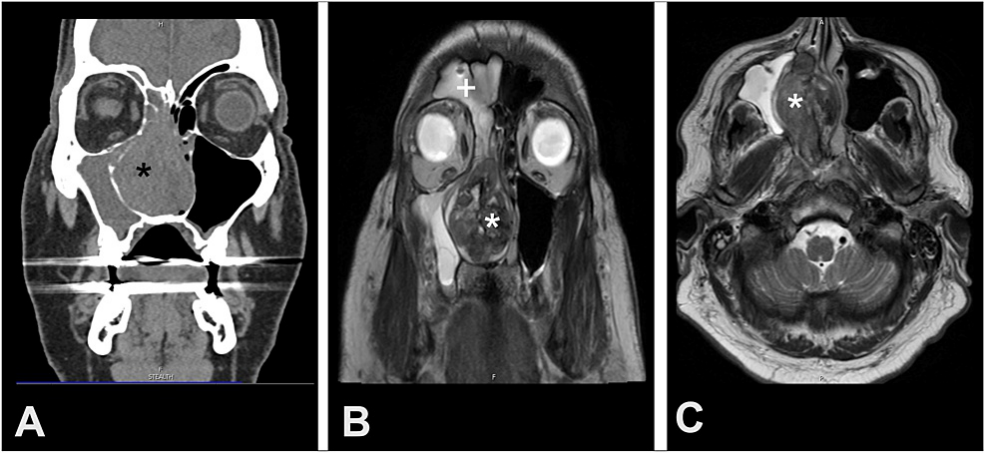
Image depicting sinus mass (*) as seen on coronal CT of head (A), image of sinus mass (*) and brain mass (+) as seen on coronal MRI of head (B), sinus mass (*) as seen on axial MRI of head (C).
